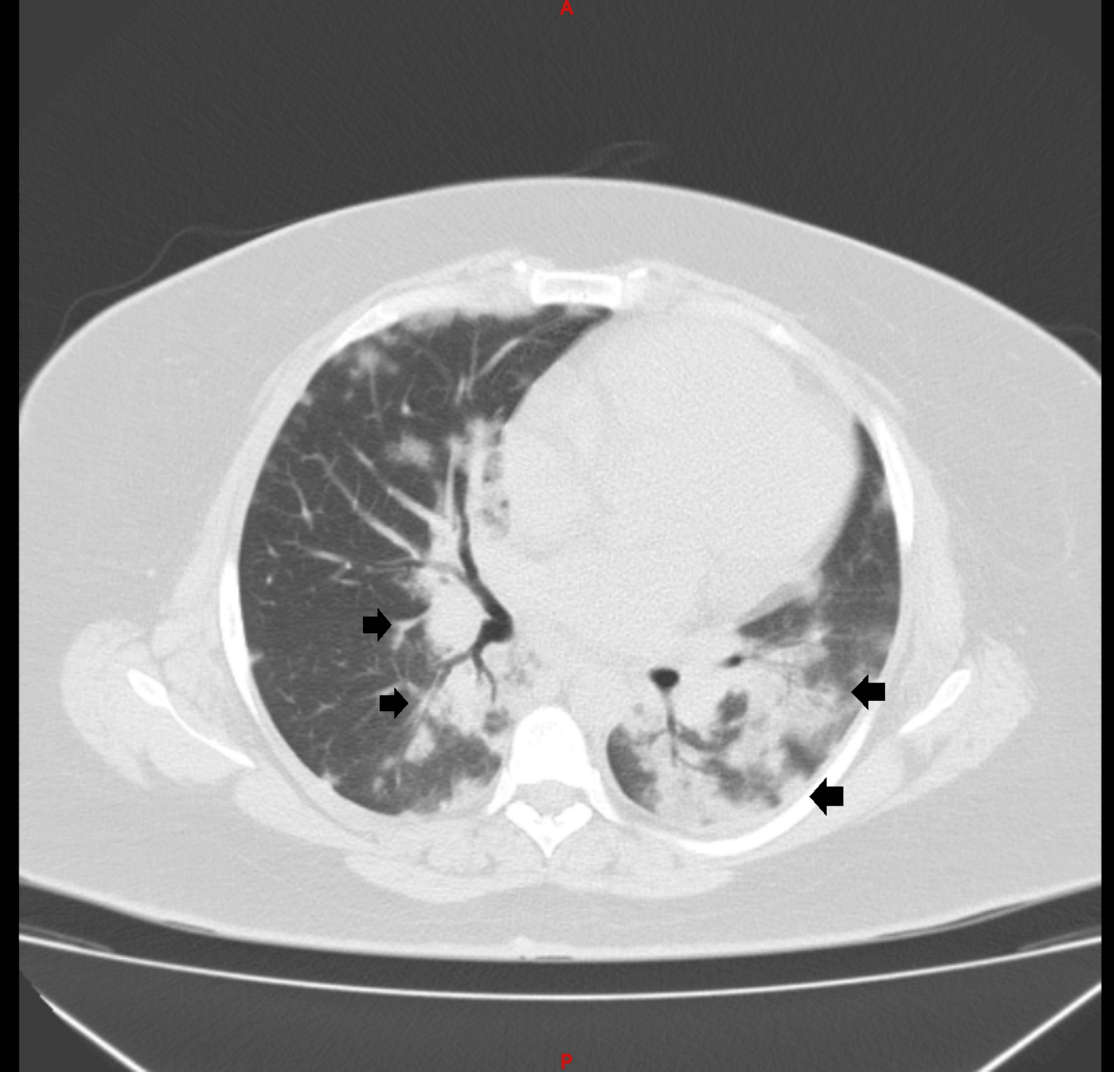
Computed tomography axial slice: air bronchogram with central and peripheral involvement, with approximately 40% of the lung parenchyma compromised. Black arrows: multiple alveolar opacities are observed in the lung parenchyma.
